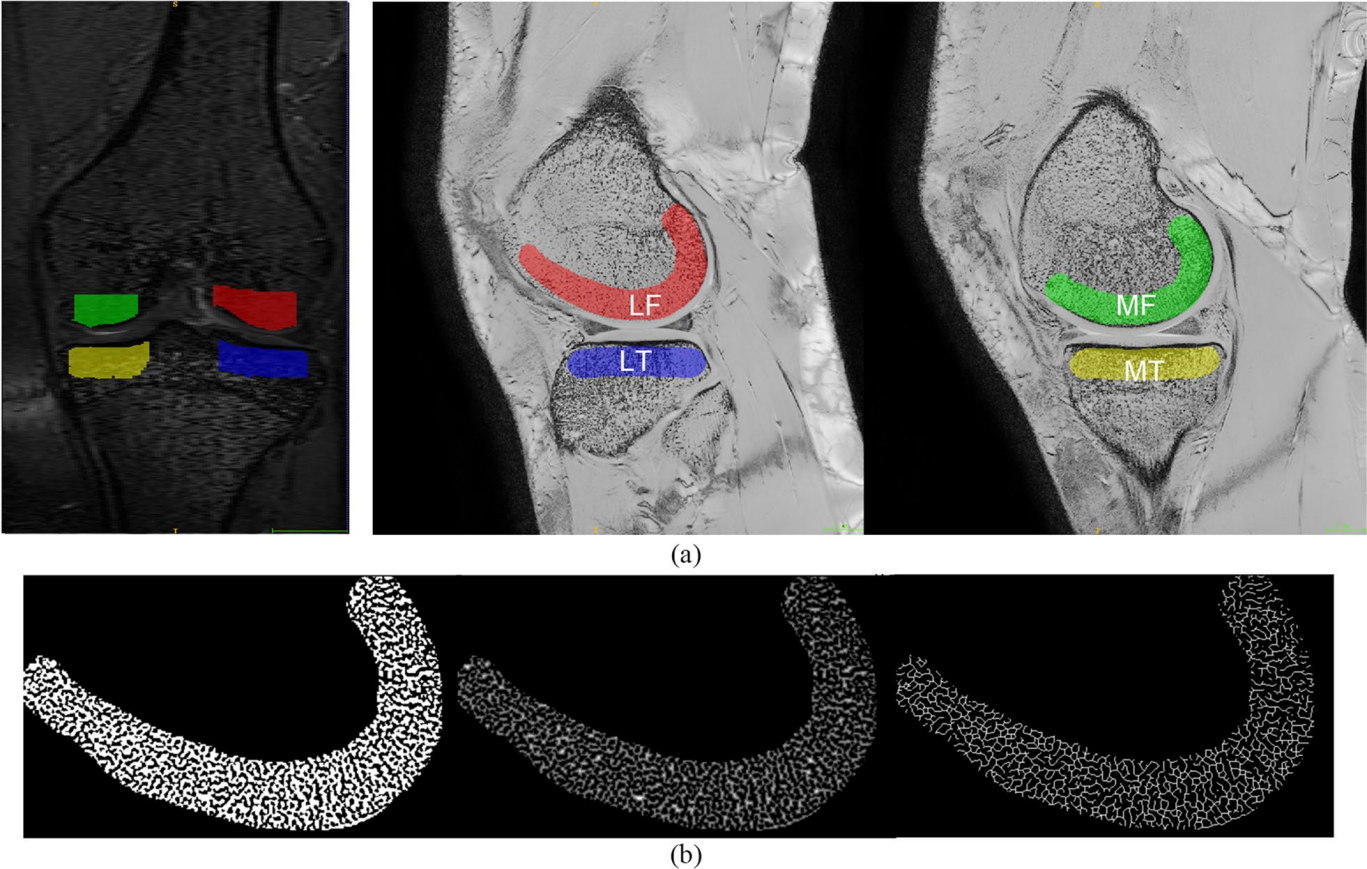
Region delineation and trabecular bone segmentation. a Four regions of interests (ROIs) were segmented from knee joint MR images named medial femoral condyle (MF), lateral femoral condyle (LF), medial tibial plateau (MT) and lateral tibial plateau (LT). b Obtained trabecular bone segmentation at the lateral femur. From left to right: binarized using adaptive threshold; after the distance transformation; after moving redundant pixels, which was obtained by multiplying the second one with the morphological skeletons
